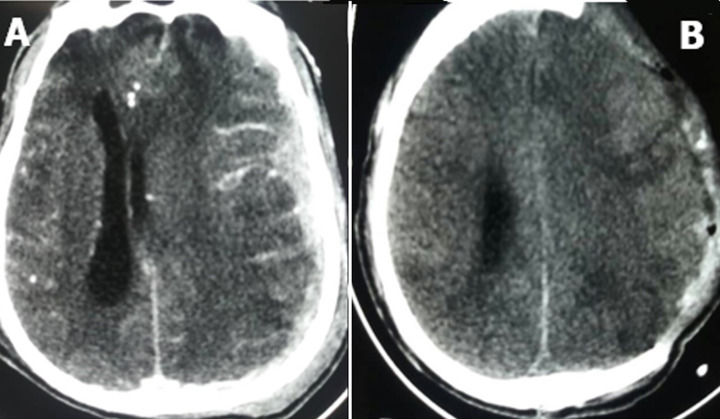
A) axial section brain CT scan showing acute subdural hematoma; B) post-operative cerebral CT scan of a decompressive craniectomy for an acute subdural hematoma in the same patient
